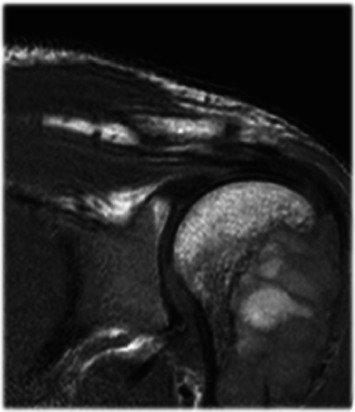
MRI of left shoulder. It shows cyst-like lesion in the proximal humeral area involving the humeral trochitis.
